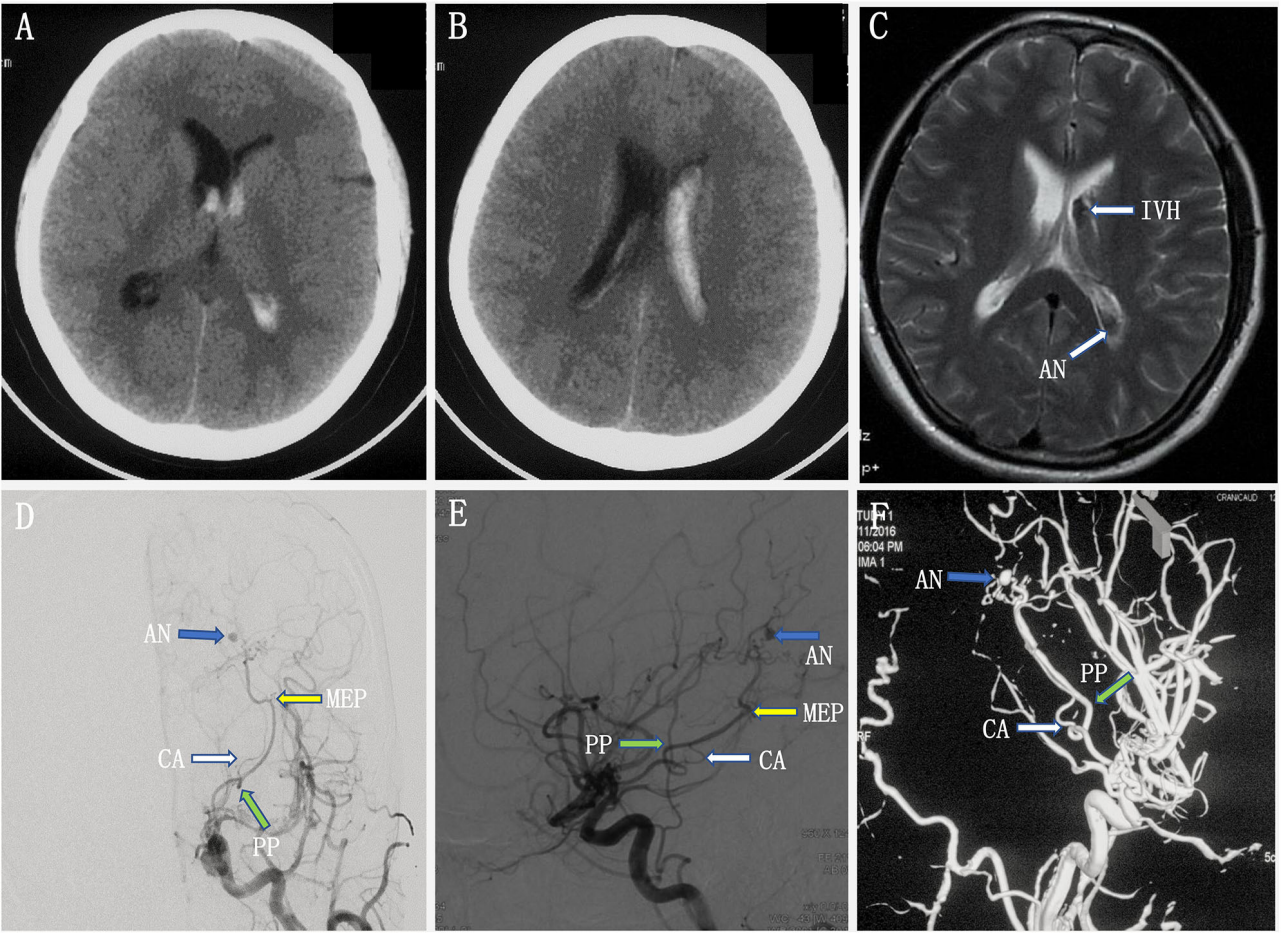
(A–C) Preoperative head computed tomography (CT) showed a left intraventricular hemorrhage, and axial magnetic resonance imaging (MRI) demonstrated a dilated artery and a suspected aneurysm in the left ventricle. (D,F) Preoperative digital subtracted angiography (DSA) [anteroposterior (AP) (D) and lateral (E) views] and 3D rotational angiographies (F) showed a narrowed internal carotid artery (ICA) bifurcation, A1, and M1 with Moyamoya change (Suzuki stage II–III). In addition, there was a dilated anterior choroidal artery with a distal aneurysm beyond the most external point (MEP).
